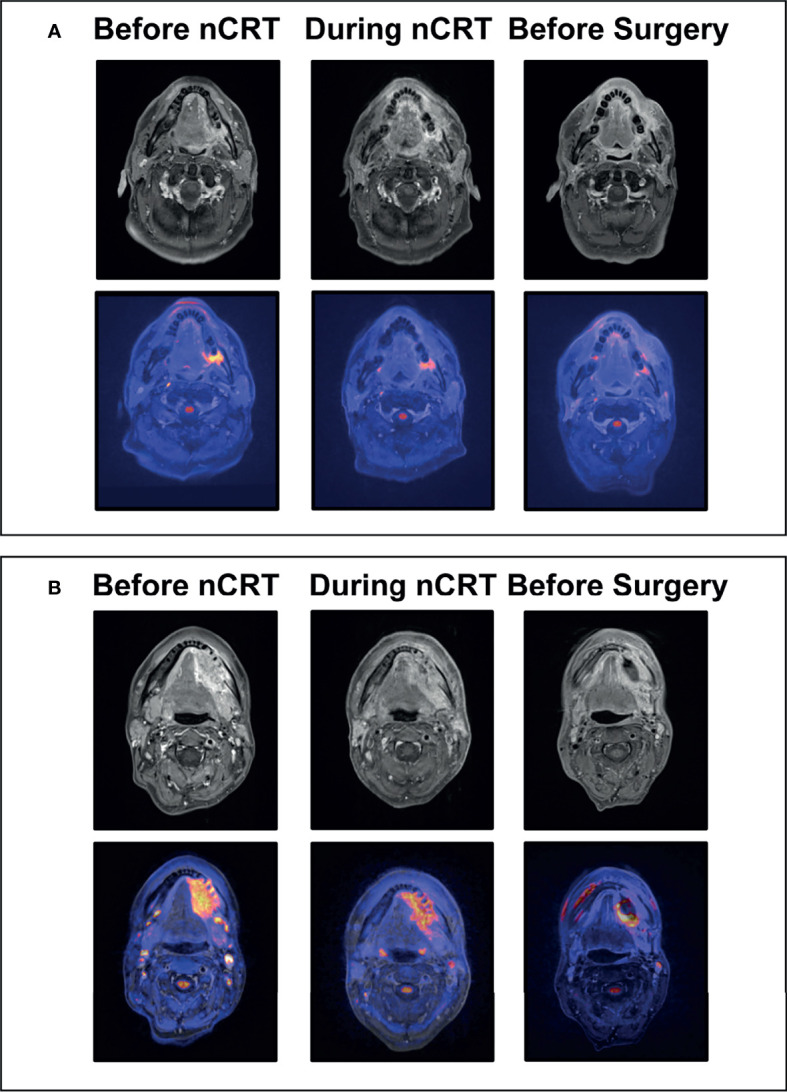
Exemplary MRI Images of Clinical Responses to Neoadjuvant Chemoradiotherapy. (A) Exemplary images of a 55-year old patient with left-sided squamous cell carcinoma of the oral cavity before and during chemoradiotherapy (day 15), and prior to surgery; The top row shows representative axial gadolineum-enhanced T1-weighted images with continuous decrease in size and contrast enhancement resulting in complete clinical response prior to surgery of the primary tumor at the left retromolar region; The bottom row shows corresponding fused diffusion-weighted - gadolineum-enhanced T1-weighted images with decreasing diffusion restriction of the tumor region resulting in complete clinical response prior to surgery. (B) Exemplary images of a 49-year old patient with left-sided squamous cell carcinoma of the oral cavity before and during chemoradiotherapy (day 15), and prior to surgery; The top row shows representative axial gadolineum-enhanced T1-weighted images with continuous decrease in size and contrast enhancement. Markable residual tumor with contrast enhancement at the left mandibular region prior to surgery; The bottom row shows corresponding fused diffusion-weighted - gadolineum-enhanced T1-weighted images with decreasing but residual diffusion restriction of the tumor region; nCRT, Neoadjuvant chemoradiotherapy.
